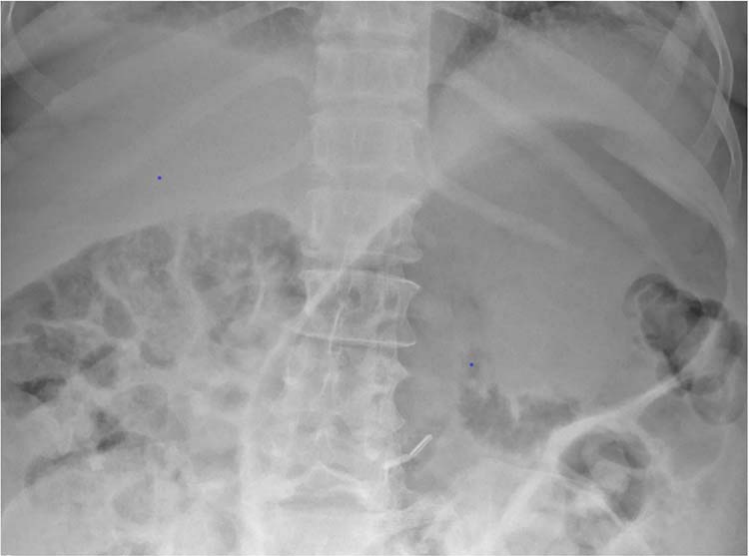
Plain film radiograph of the abdomen demonstrating a distended and gas-filled stomach.
